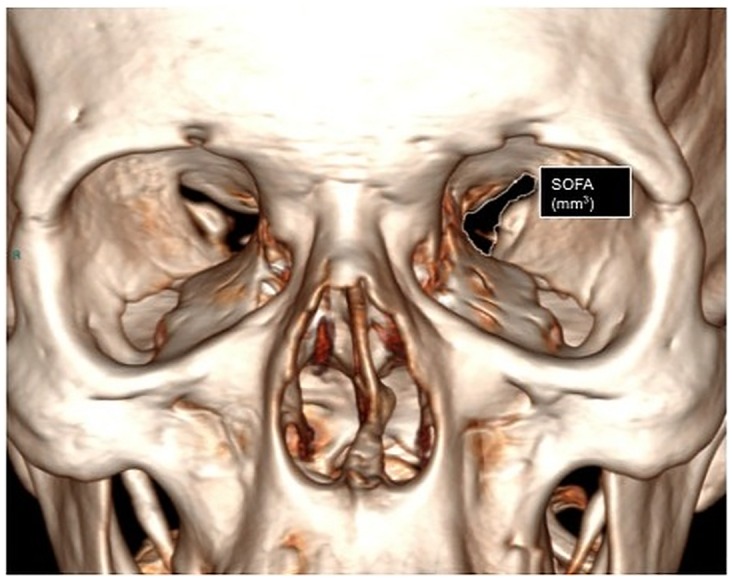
3D CT reconstruction. Measurements of the superior orbital fissure area obtained using curve measurement (SOFA).
